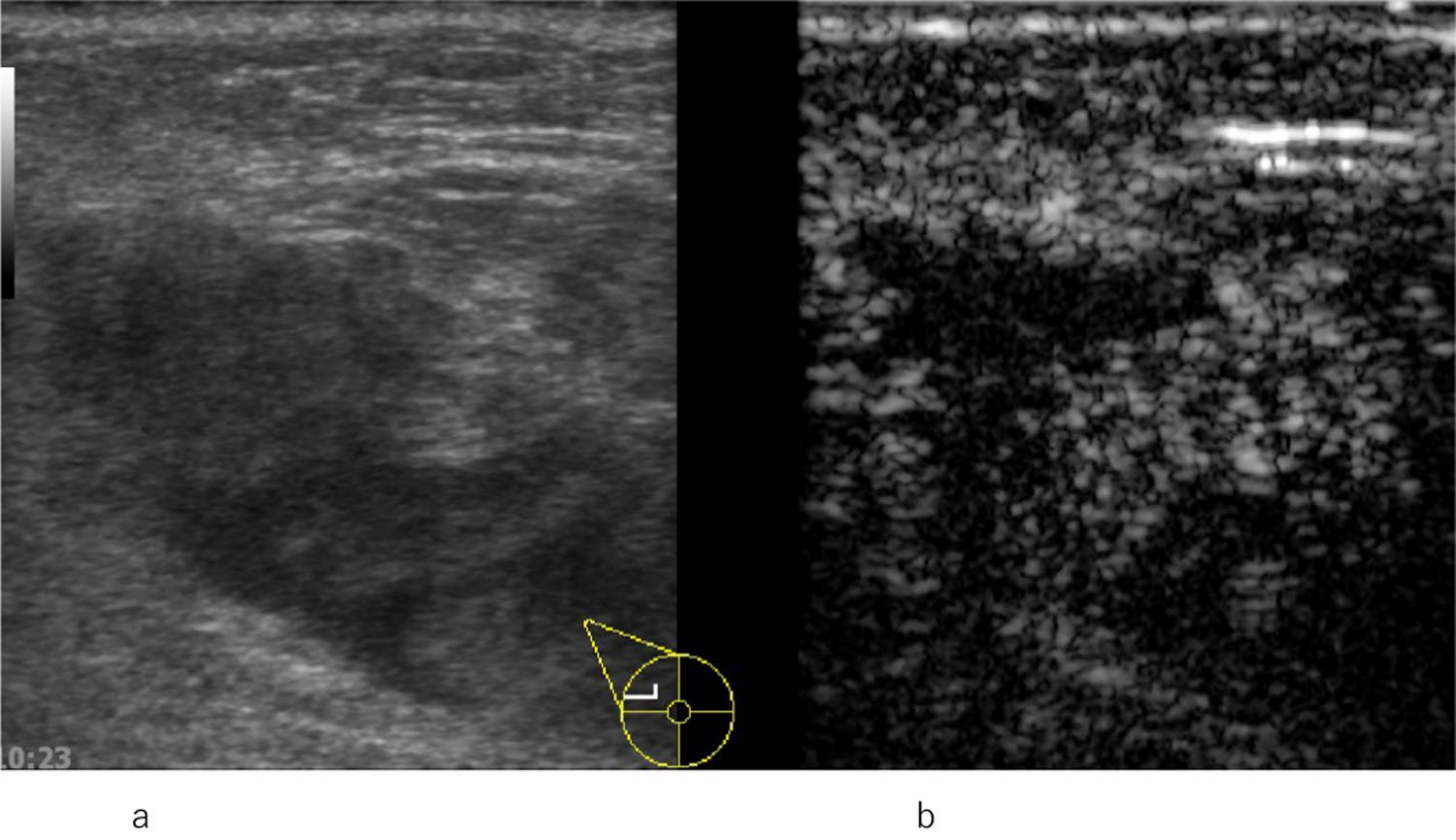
Invasive ductal carcinoma. a Conventional ultrasound shows a hypoechoic area. b Contrast-enhanced ultrasound shows heterogeneous enhancement with a clear defect
